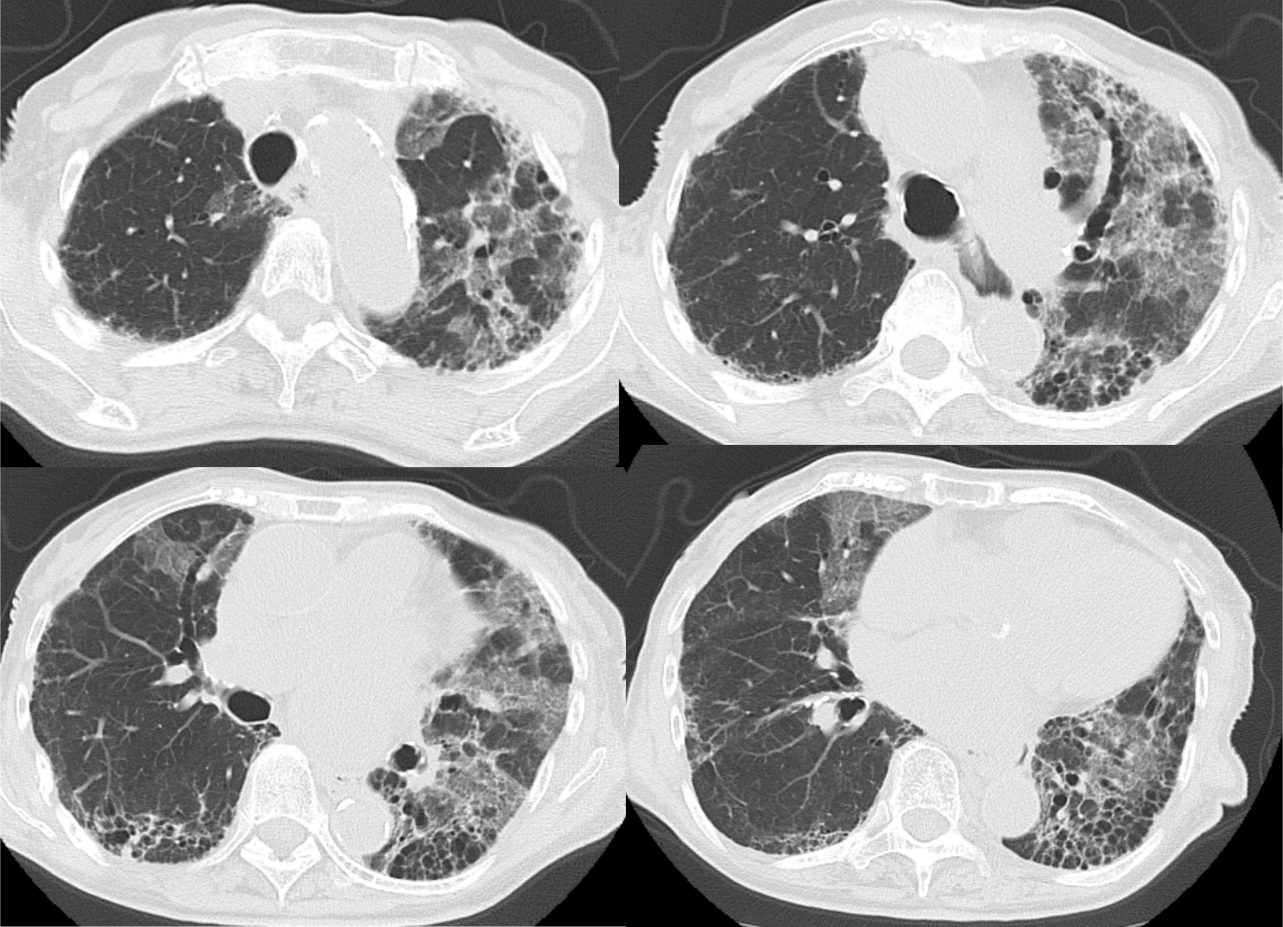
High‐resolution chest computed tomography (HRCT) image at the time of admission. The scan shows bilateral ground‐glass opacities and reticular changes predominantly in the lower lung fields, consistent with a progression of pre‐existing ILD.
